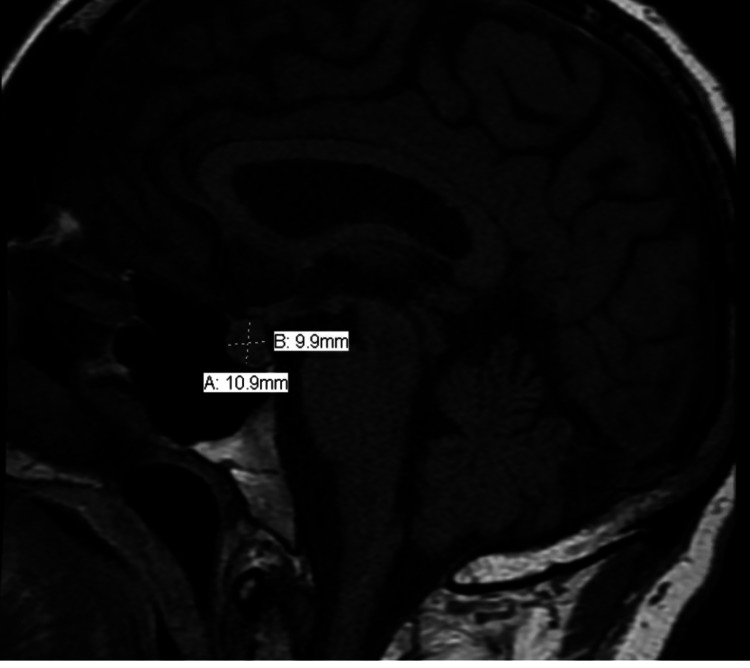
MRI brain showing pituitary gland enlargement in sagittal section MRI: magnetic resonance imaging
